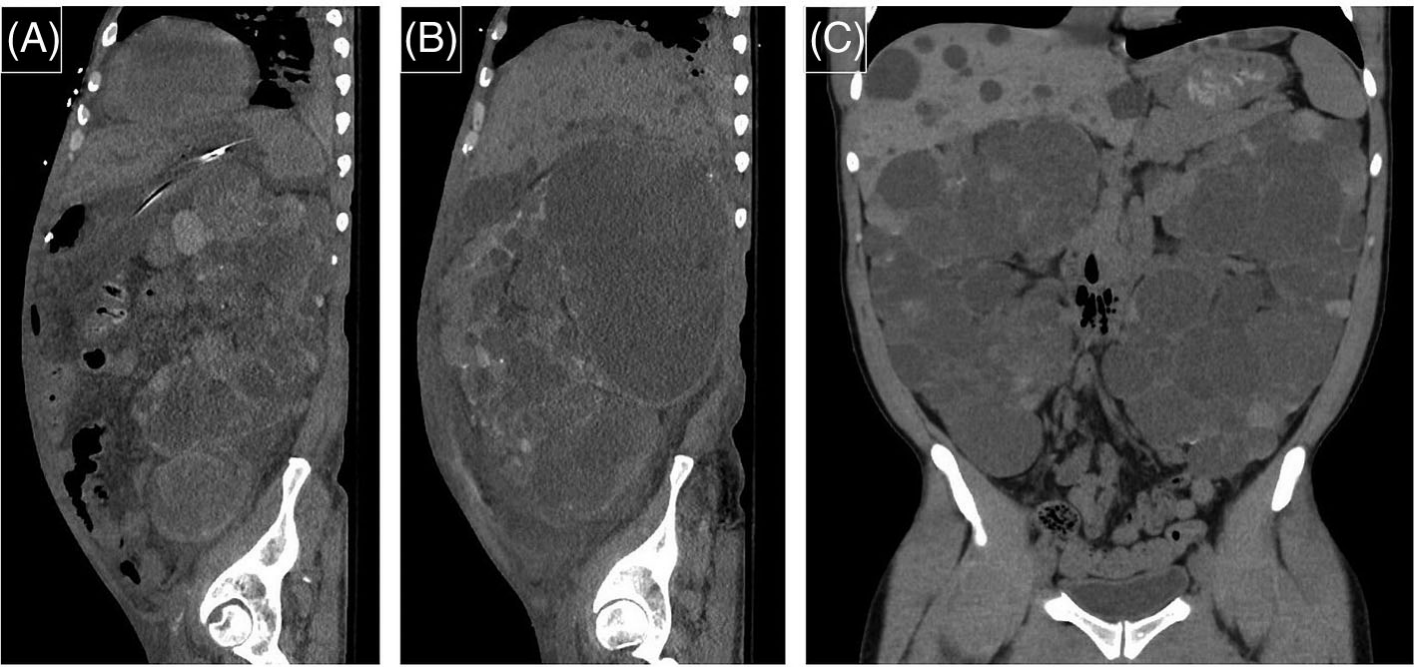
Preoperative computed tomography (CT) images of polycystic kidneys: (A) largest right kidney removed 29.3 cm craniocaudally; (B) largest left kidney removed 31.8 cm craniocaudally and (C) bilateral kidneys cross midline and nearly fill the abdominal cavity.
